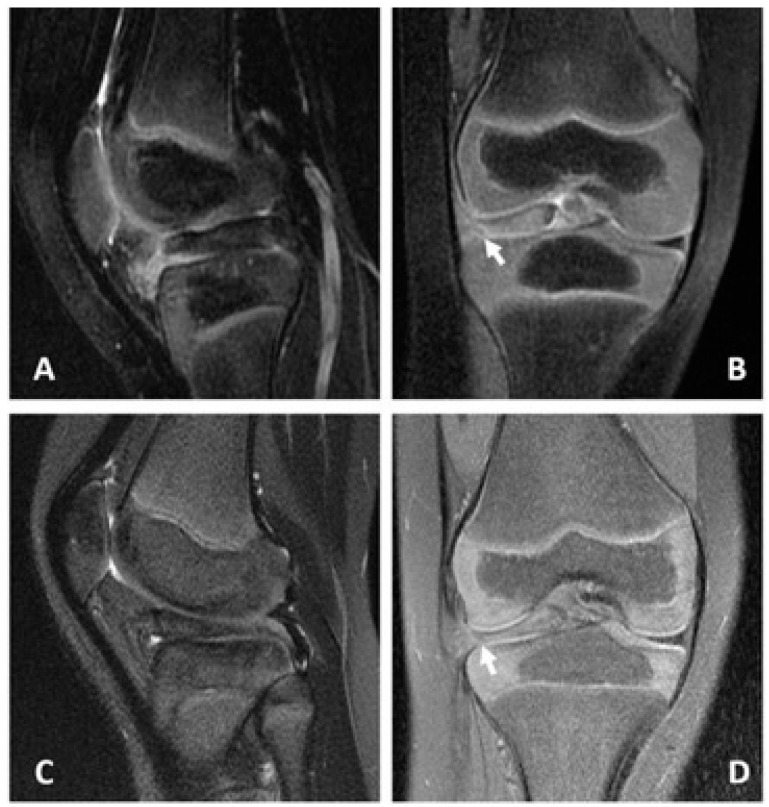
Preoperative MRI of the affected knees for the two cases. (A) coronal and (B) sagittal planes for the case of the 28-month-old girl. (C) coronal and (D) sagittal planes for the case of the 5-year-old boy. Both lateral menisci revealed increased thickness and flattening, loss of the natural bow-tie configuration, and a peripheral tear in the posterior horn. The white arrow indicates the peripheral tear site. MRI: magnetic resonance imaging.
